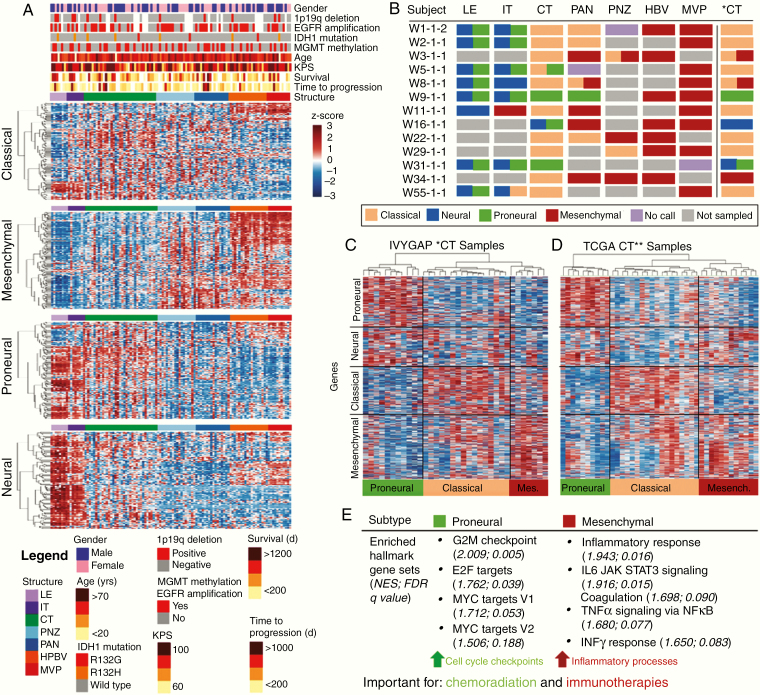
Molecular subtype classification depends on the structure sampled, with CT able to distinguish biologically distinct subtypes. (A) Expression of subtype gene sets (*y*-axis) in IvyGAP samples (*x*-axis) from each region show that sample structure is a main contributor to expression of subtype gene signatures. Genes corresponding to each subtype were organized independently by unsupervised hierarchical clustering. (B) Subtype classification for samples from subjects with data from ≥4 different regions. CT* represents subtype analysis applying data *z*-scored across only CT samples. (C) Unsupervised hierarchical clustering of IvyGAP CT samples (data *z*-scored across CT samples) showing 3 main clusters with signatures of proneural, classical, and mesenchymal subtypes. (D) Unsupervised hierarchical clustering of TCGA CT-classified samples demonstrating 3 main clusters with signatures of proneural, classical, and mesenchymal subtypes. CT represents TCGA samples predicted to be composed of predominantly CT based on our structure prediction gene signature. (E) Enrichment of hallmark gene sets in proneural and mesenchymal subtypes, characterized based on CT sample analysis. Proneural and mesenchymal tumors have enrichment of cell cycle checkpoints and immune processes, respectively. FDR, false discovery rate; NES, normalized enrichment score.
